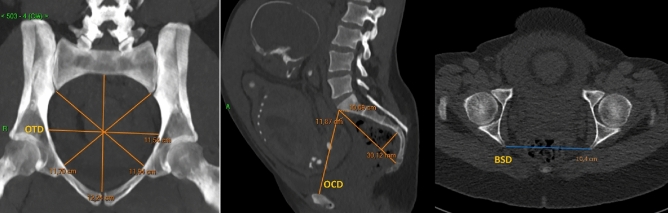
Main pelvic diameters on a CT pelvimetry. *OTD* Obstetric transverse diameter, *OCD* Obstetric conjugate diameter, *BSD* Bispinous Diameter, *CM* centimeters.
